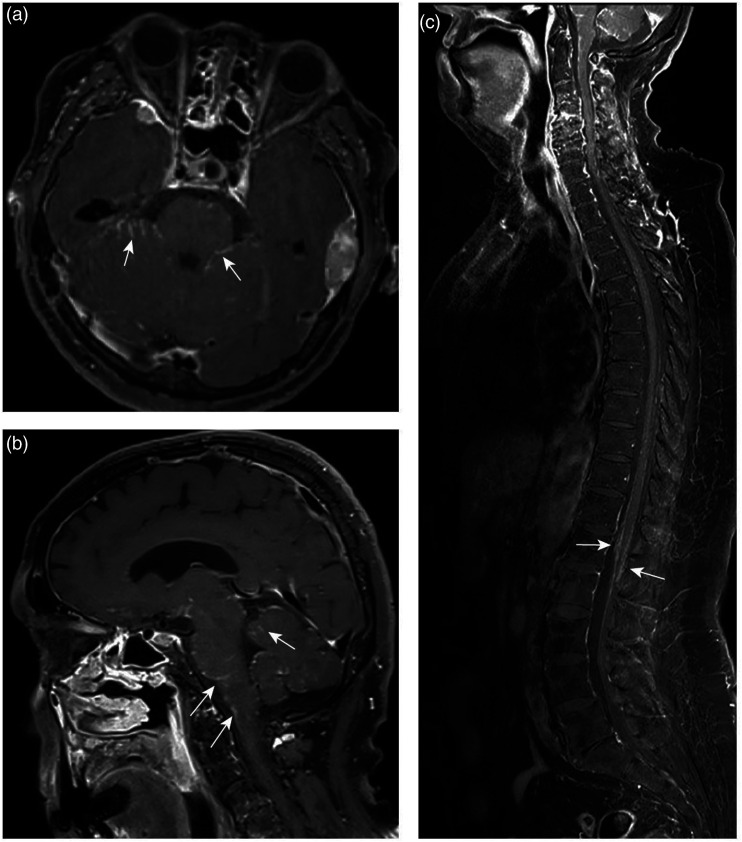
Axial and sagittal, postgadolinium T1-weighted MRI of the head reveals subtle, nodular thickening and enhancement of the leptomeninges, most pronounced infratentorial (A, B, white arrows). Sagittal, postgadolinium *water-only* T1 Dixon MRI of the whole spine shows leptomeningeal enhancement along the spinal cord and cauda equina (C, white arrows).
